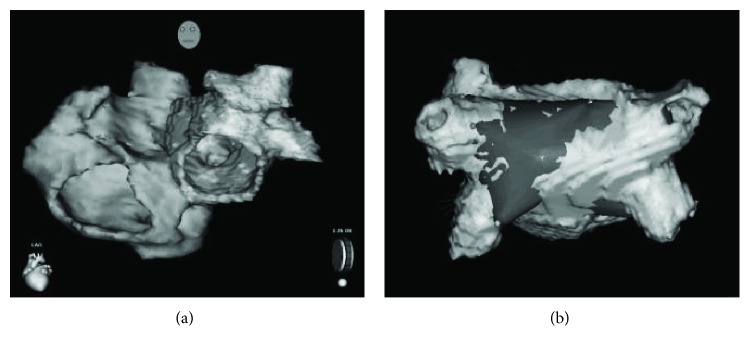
(a) CT-derived reconstruction of the LA chamber of the mock silicone model; (b) the reconstructed CARTO map (dark grey area) merged to CT reconstruction.
